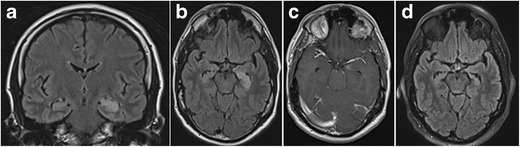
Limbic encephalitis. 1.5-T coronal FLAIR (a), axial FLAIR (b) and contrast-enhanced T1W images (c) show T2-FLAIR-hyperintense swelling of the left hippocampus and discrete patchy enhancement in the left amygdala. On axial FLAIR on follow-up MRI performed 6 months later (d), the signal abnormalities have disappeared and there is volume loss of the left hippocampus with loss of the normal hippocampal digitations of the hippocampal head
